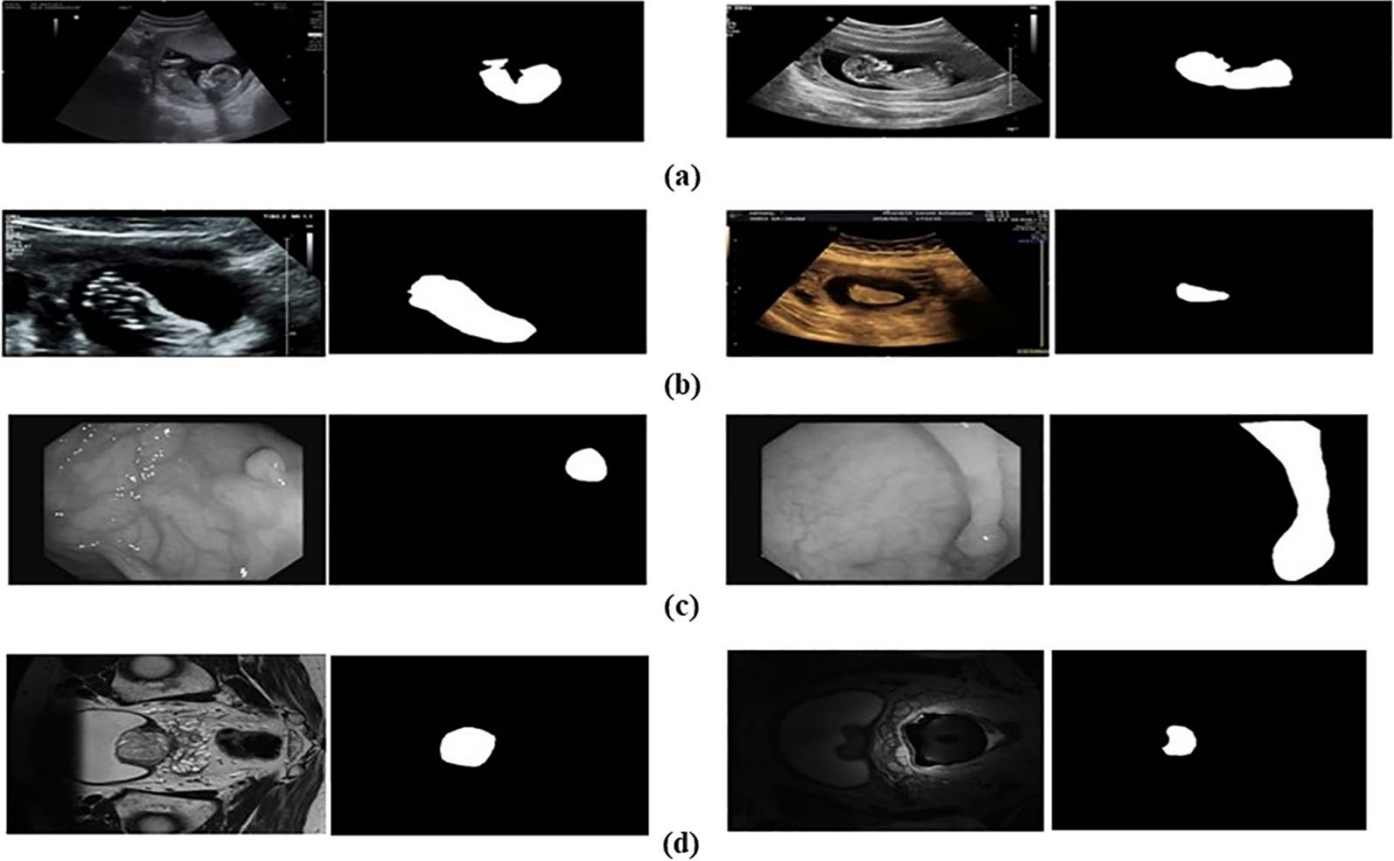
Samples of the CRL Foot-MFP, CVC-ClinicDB, and multi-site MRI datasets together with their corresponding annotation of the target structures. CRL (a), Fetal Foot (b), CVC-ClinicDB (c), Multi-site MRI (d).
